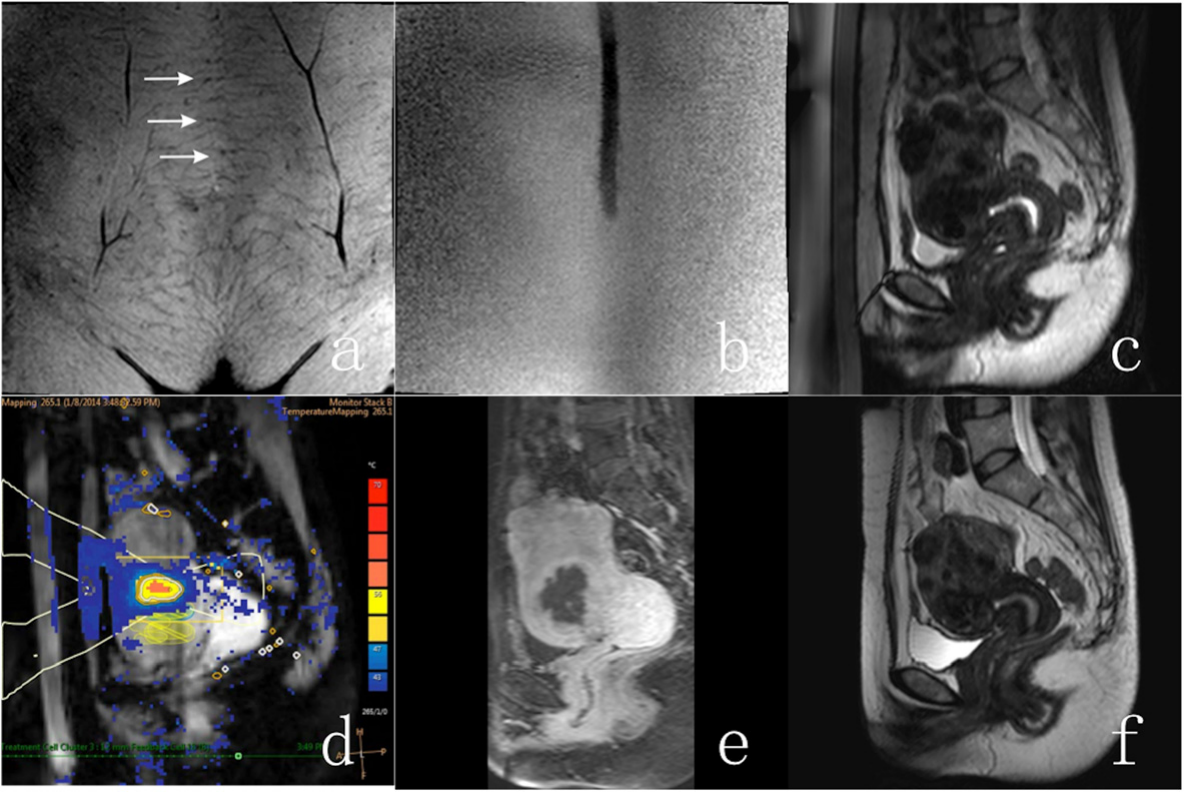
A 49-year-old woman who had a longitudinal incision presented with symptoms of menorrhagia, pelvic pressure, and urinary frequency was treated with MRI-guided high-intensity focused ultrasound ablation using scar patch. a Scar scan showing the orientation of the scar within the abdominal fat layer (scar location identified with white arrows). b Scar scan showing the air-containing scar patch at the patient’s skin. c T2w planning image acquired prior to the therapy. d PRF Thermometry scan acquired during one of the sonications. e T1w gadolinium-enhanced image acquired immediately after the therapy showing the induced necrosis. f T2w planning image at 6-month follow-up
